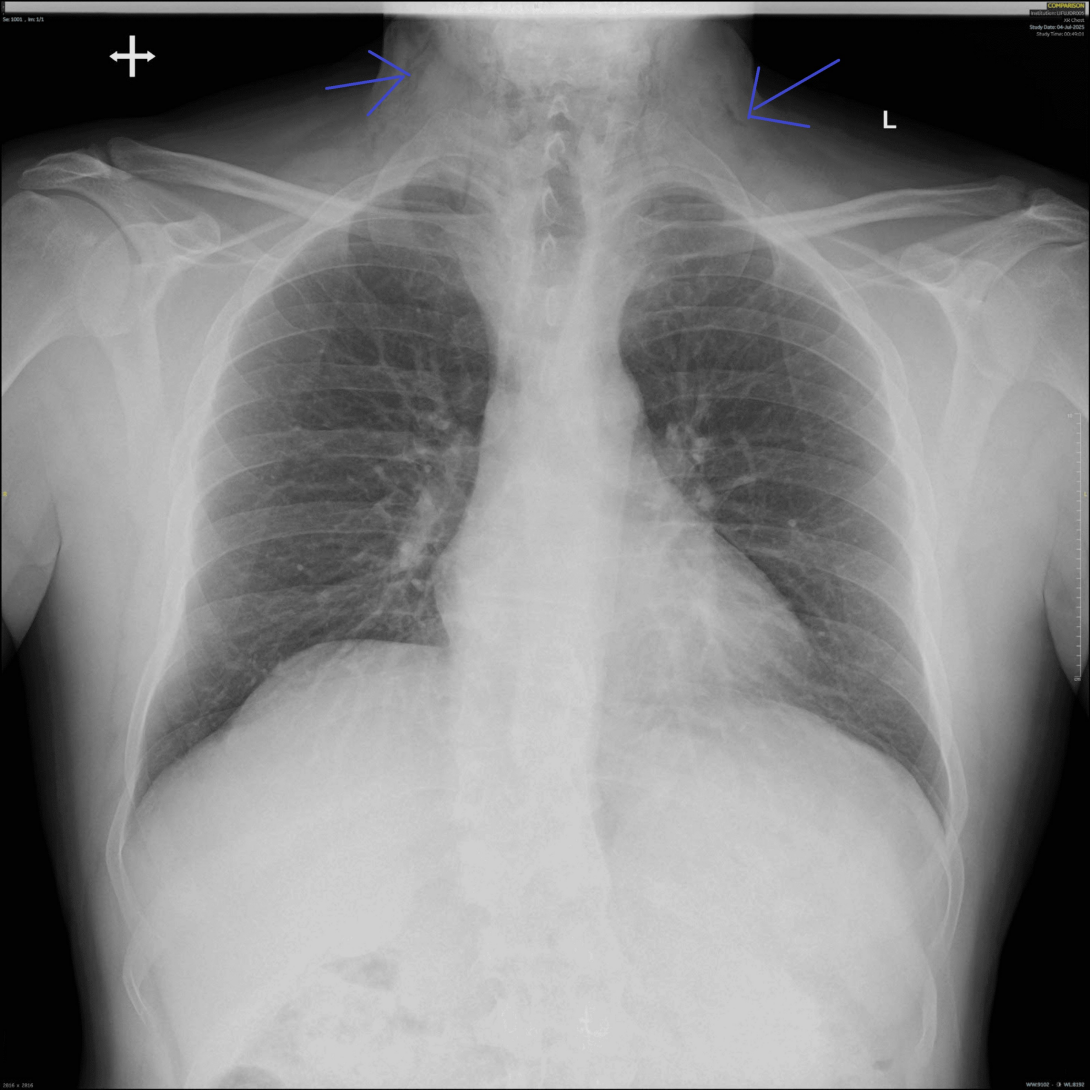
Posteroanterior chest x-ray/lateral soft tissue neck view Extensive surgical emphysema noted within the upper mediastinum along with soft tissues of the neck. No evidence of retained foreign body within the prevertebral soft tissues. Appearances raise the suspicion of an oesophageal perforation.
